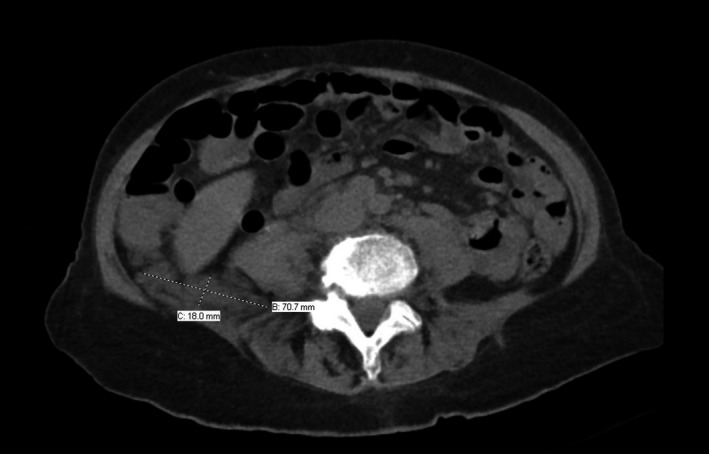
CT scan showing fluid collection and psoas abscess
